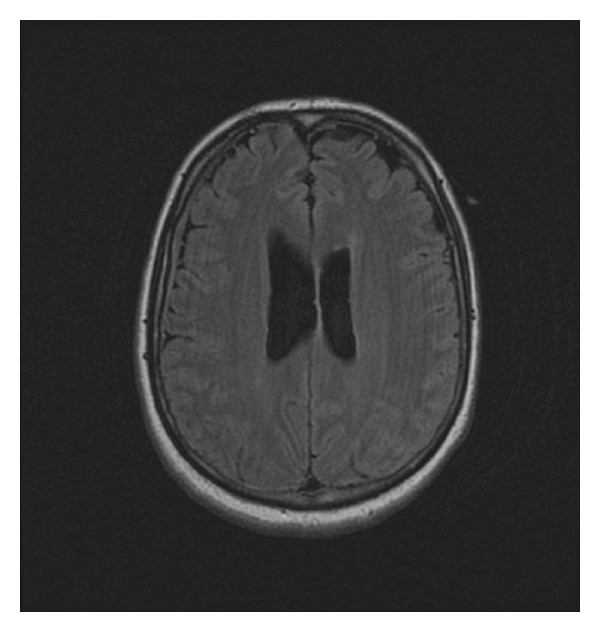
Cranial MRI image showing thin subdural hematoma in the right frontotemporal region.
